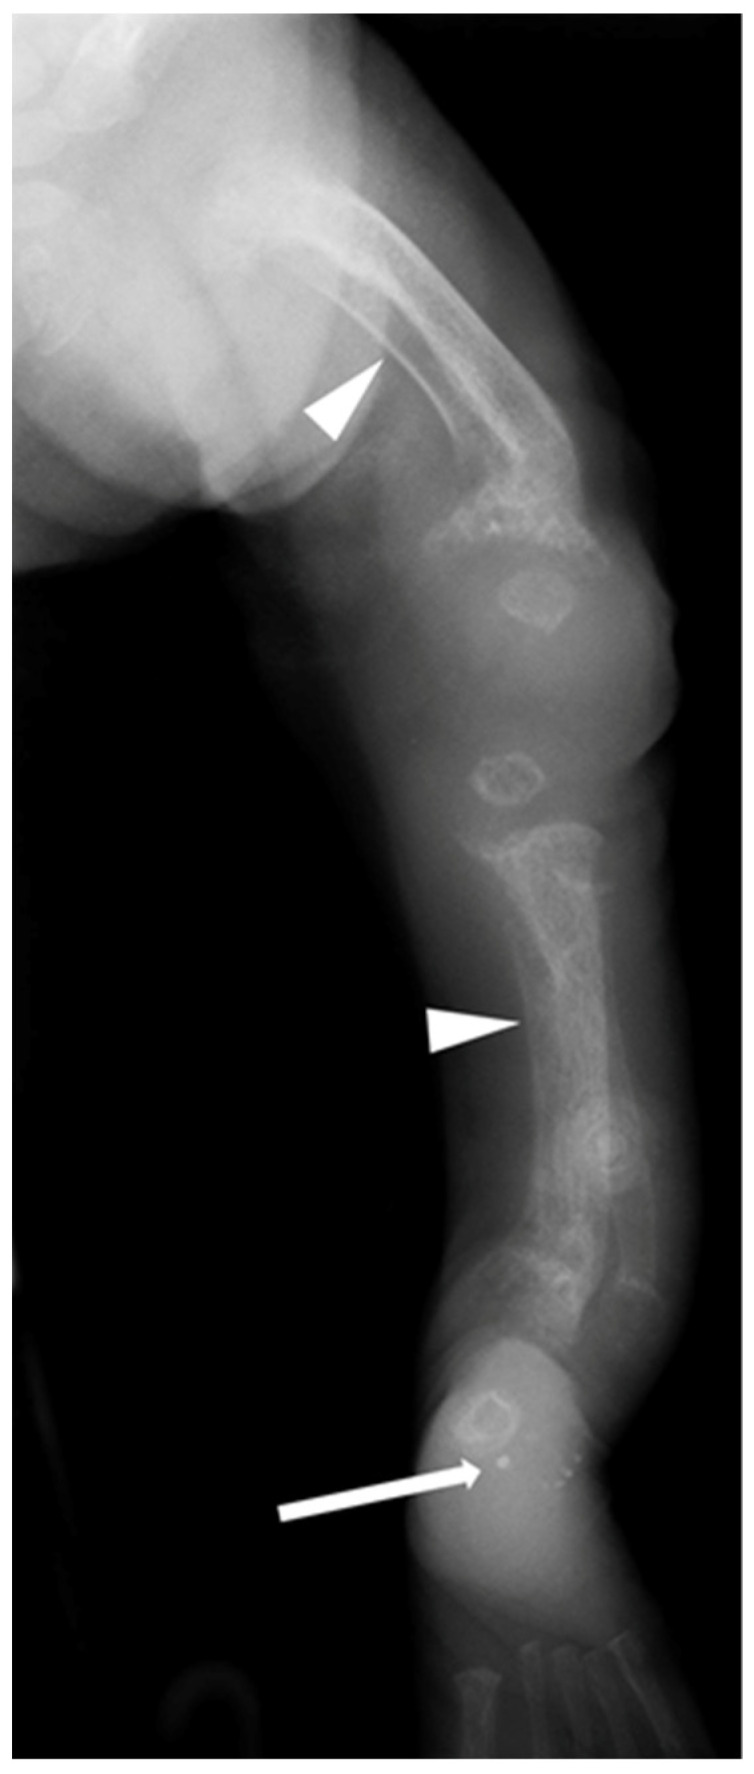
Acute findings of hyperparathyroidism and rickets. Patient 1, left leg radiograph day 1 demonstrates features of hyperparathyroidism including reduced bone density, subperiosteal resorption and poor cortical delineation (see medial tibia) as well as diaphyseal cloaking of the femur and tibia (arrowheads). Features of rickets are seen with cupped, splayed and frayed metaphyses especially in the distal femur. Diaphyseal angulation consistent with antenatal fractures is seen in the distal femur and tibia. Additionally, talocalcaneal stippling, a feature of I-cell disease is also present (white arrow).
